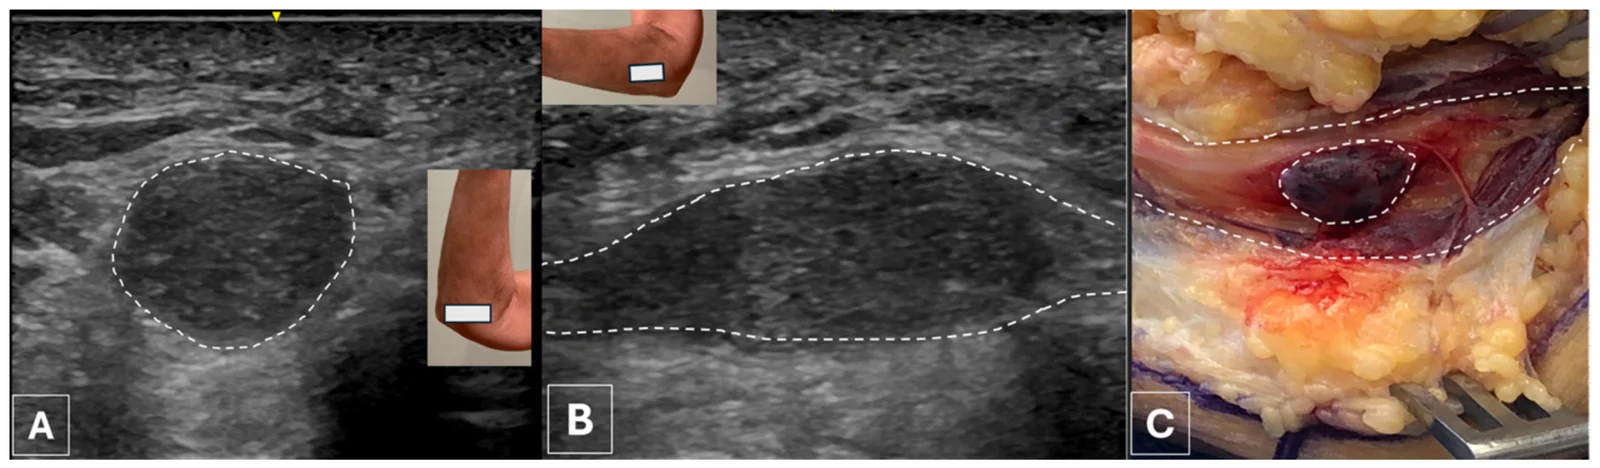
Case #4: (A) Ultrasound short-axis view at the elbow showing a markedly enlarged ulnar nerve (dotted line). (B) Long-axis view at the elbow showing an oblong mass (dotted line) in the ulnar nerve. (C). Intraoperative photo showing a Schwannoma (outer dotted line) with hemorrhage (inner dotted line) inside the mass.
